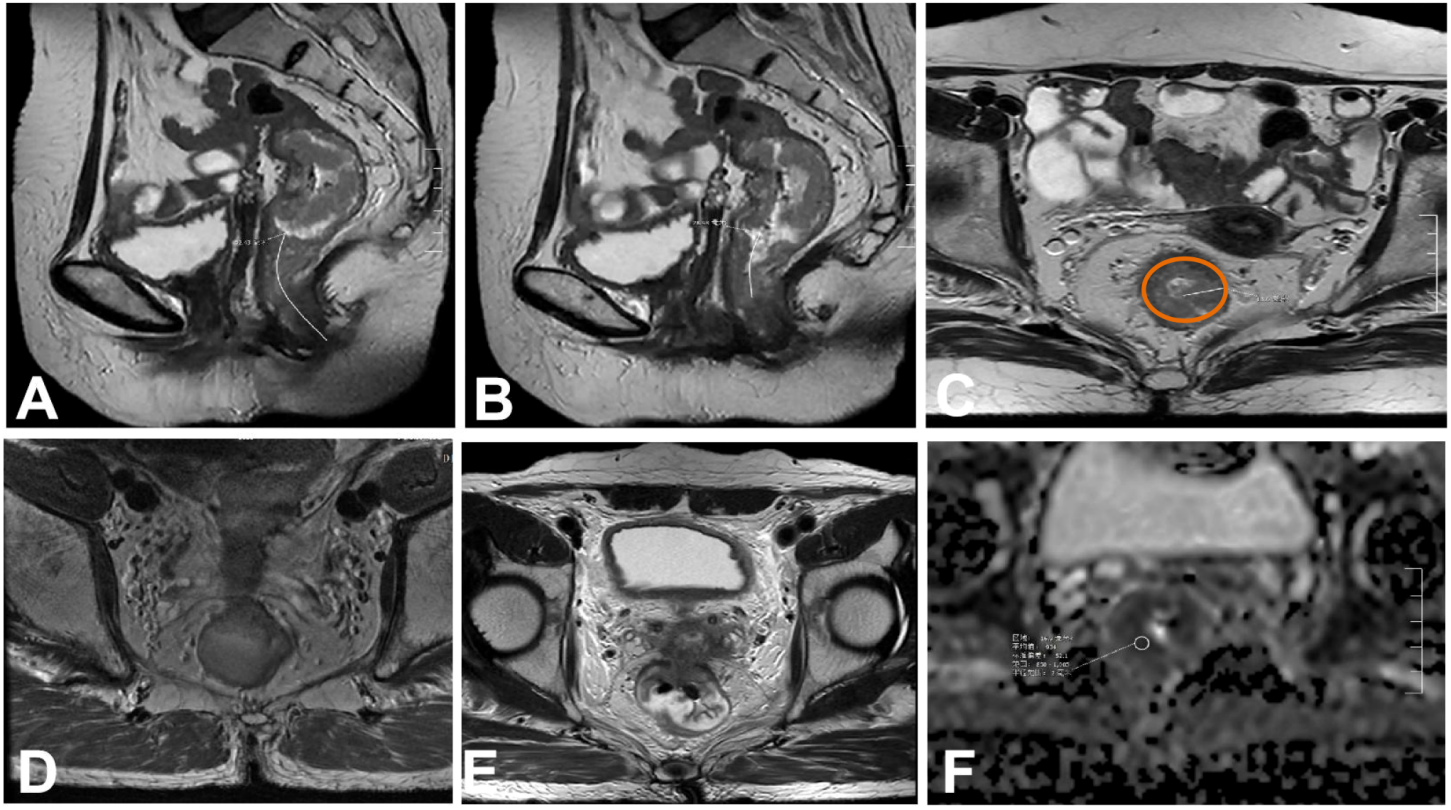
Tumor morphology detected on magnetic resonance images. (A) Distance from the lower margin of the tumor to the anal margin. (B) Distance between the lower tumor margin and the anorectal ring. (C) Maximum tumor diameter.The brown outline identify of the intestinal wall. (D) Proportion of the tumor in relation to the circumference of the intestinal wall. (E) Involvement of the circumferential resection margin. (F) apparent diffusion coeffificient (ADC) value of the lesion was measured.
